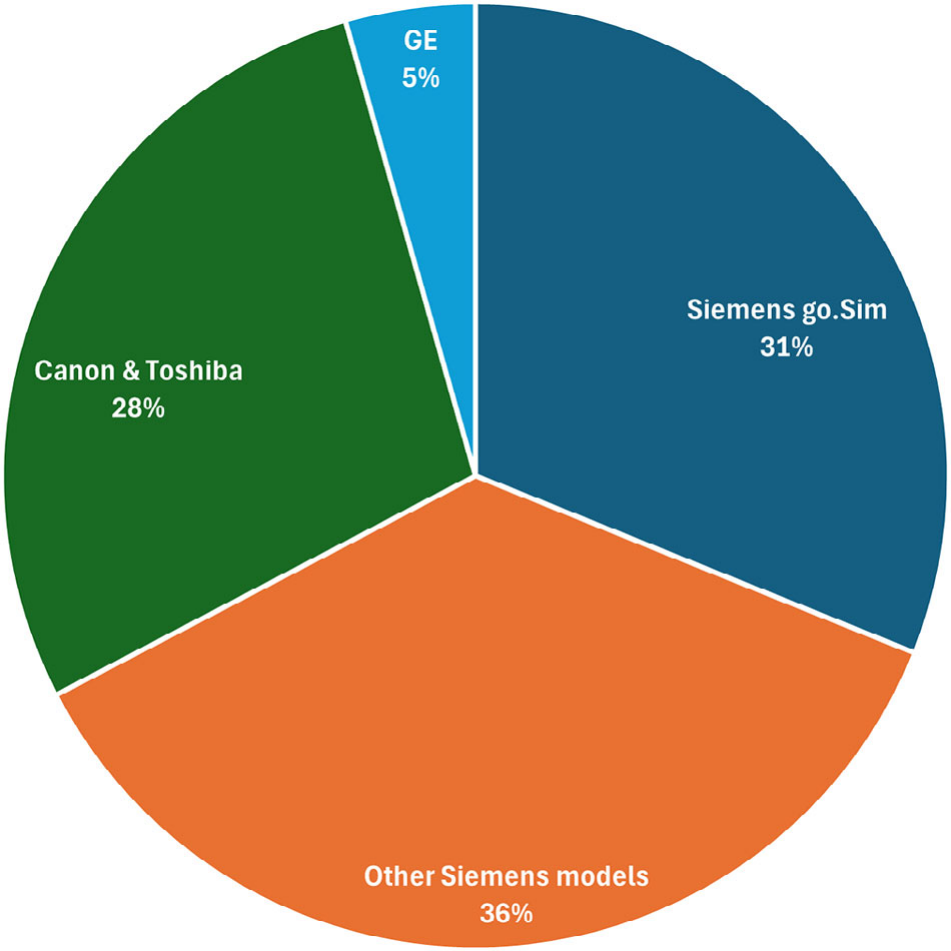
Distribution of CT datasets by scanner model.
